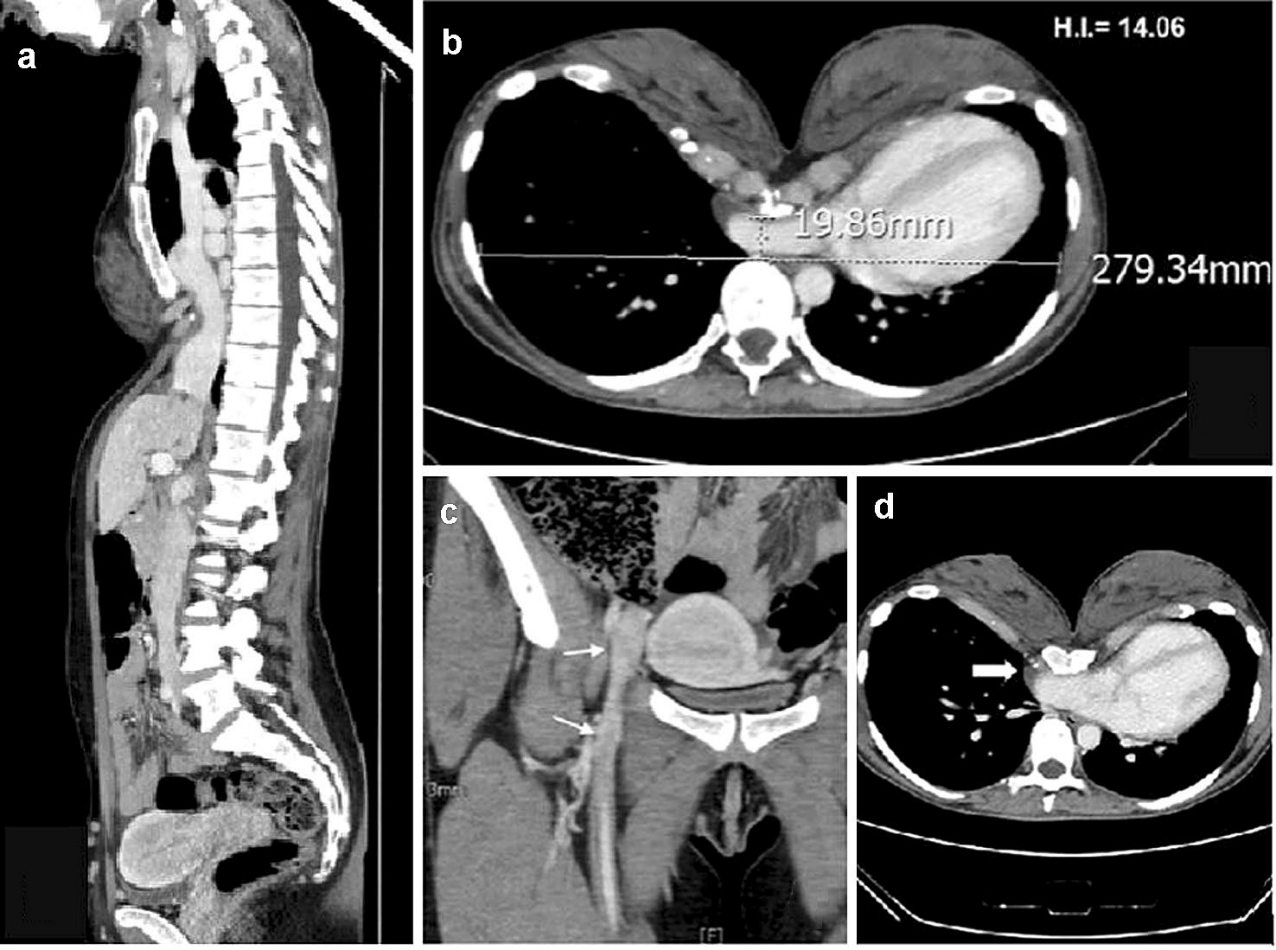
a Preoperative sagittal CT scan showing the deep depression of the sternal corpus. b Preoperative axial CT scan with Haller Index. c Preoperative coronal CT scan showing thrombosis in the deep femoral system. d Preoperative coronal CT scan showing thrombosis in the inferior vena cava
